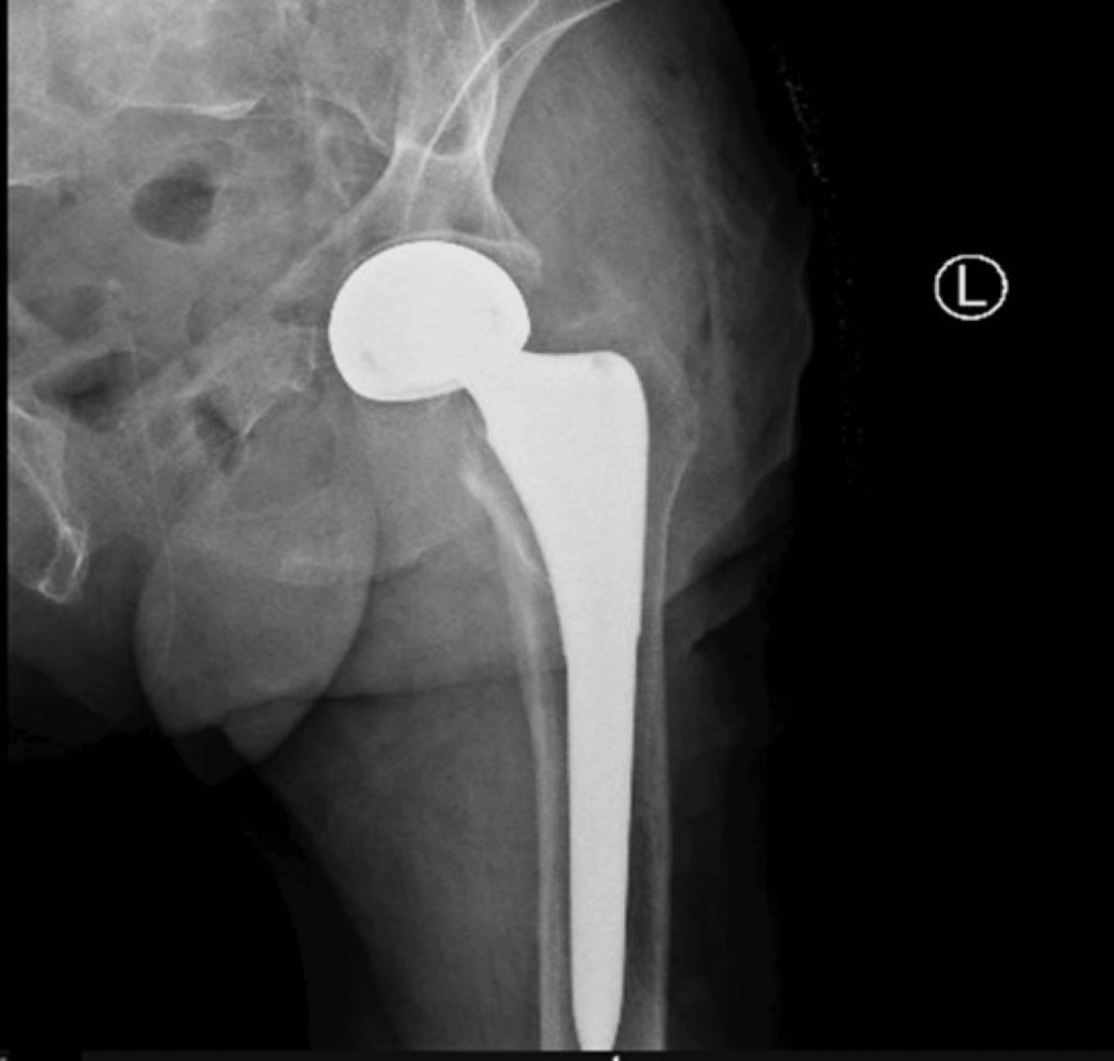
Postoperative anteroposterior X-ray
